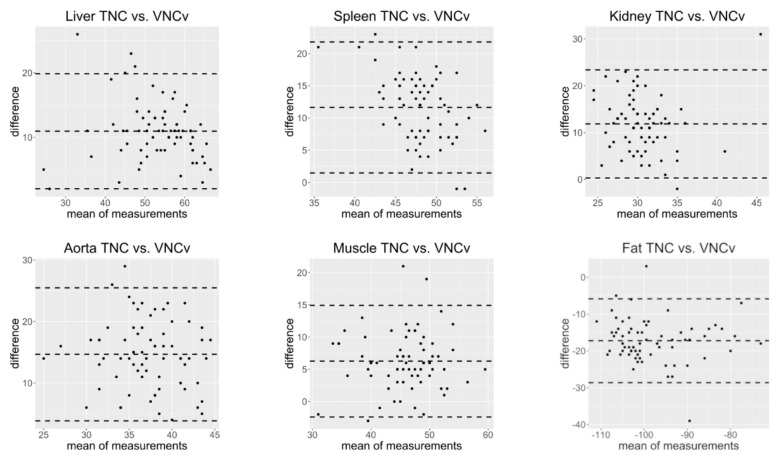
Bland Altman plots showing the relationship between the difference of measurements on true non-contrast (TNC) and virtual non-contrast images from a CT scan in venous contrast phase (VNCv) and the corresponding mean of measurements for the different tissues. All measurements spread around the mean of the differences and most measurements lie between ± 1.96 standard deviations of the difference. The difference is not substantially affected by the mean of measurements. For liver, spleen, kidney, aorta and muscle almost all measurements show a difference of >0 while for fat almost all measurements show a difference of <0.
